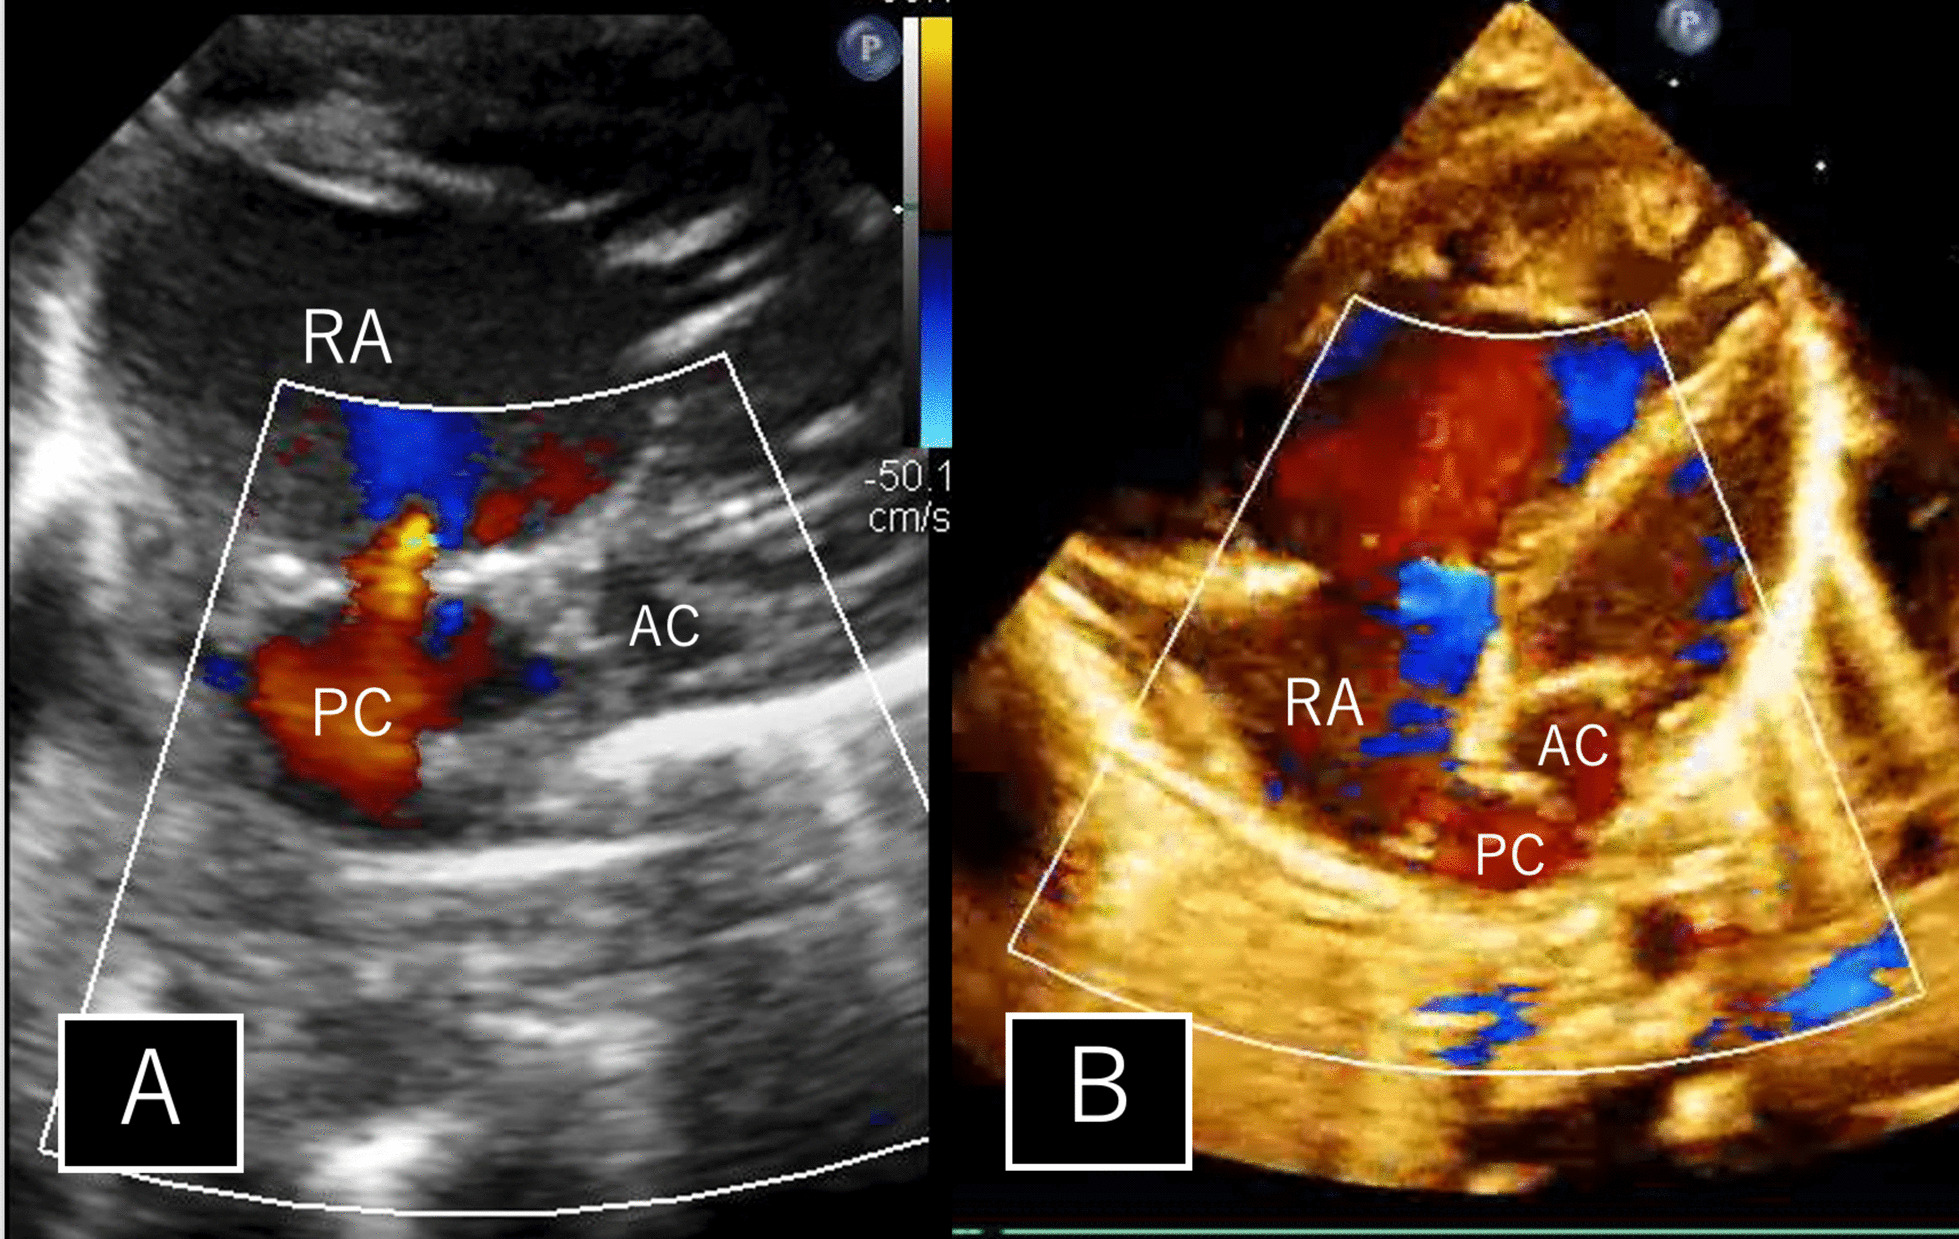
2D Doppler transthoracic echocardiogram images in patients 1 (A) and 2 (B). PC, posterior left atrial chamber; AC, anterior left atrial chamber; RA, right atrium
